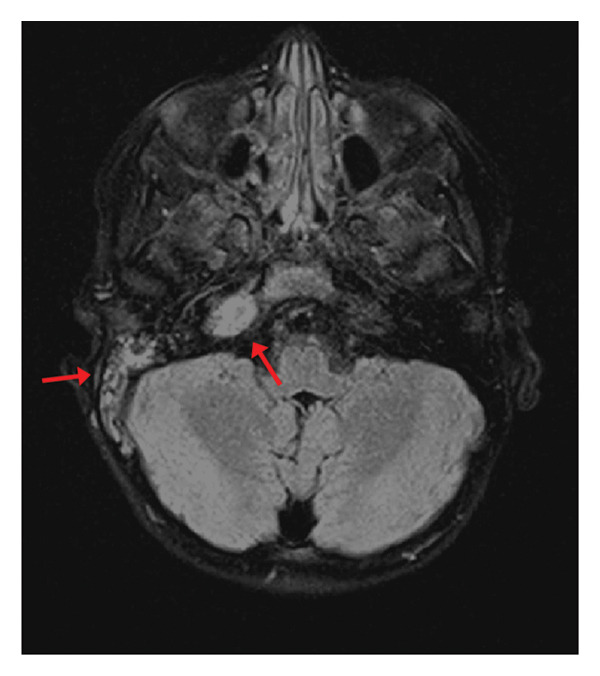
MRI brain on admission demonstrating right mastoid enhancement and notable coalescent purulent contents within the petrous apex, concerning for right mastoiditis and right petrous apicitis. No pathologic brain parenchymal signal or enhancement and no convincing evidence for meningitis were seen.
